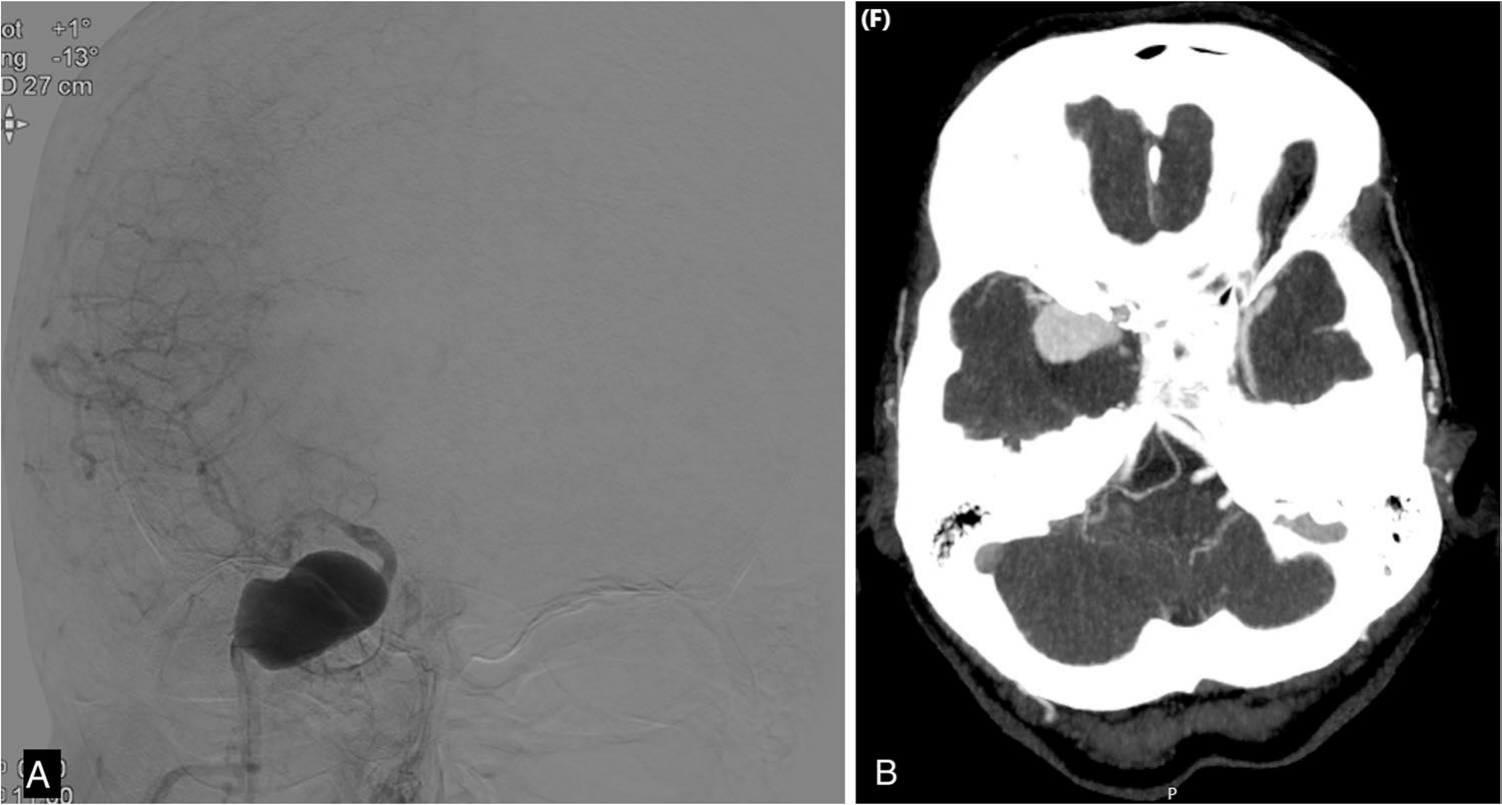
Patient 3: a: DSA of partially thrombosed of aneurysm of right internal carotid artery b: CTA
